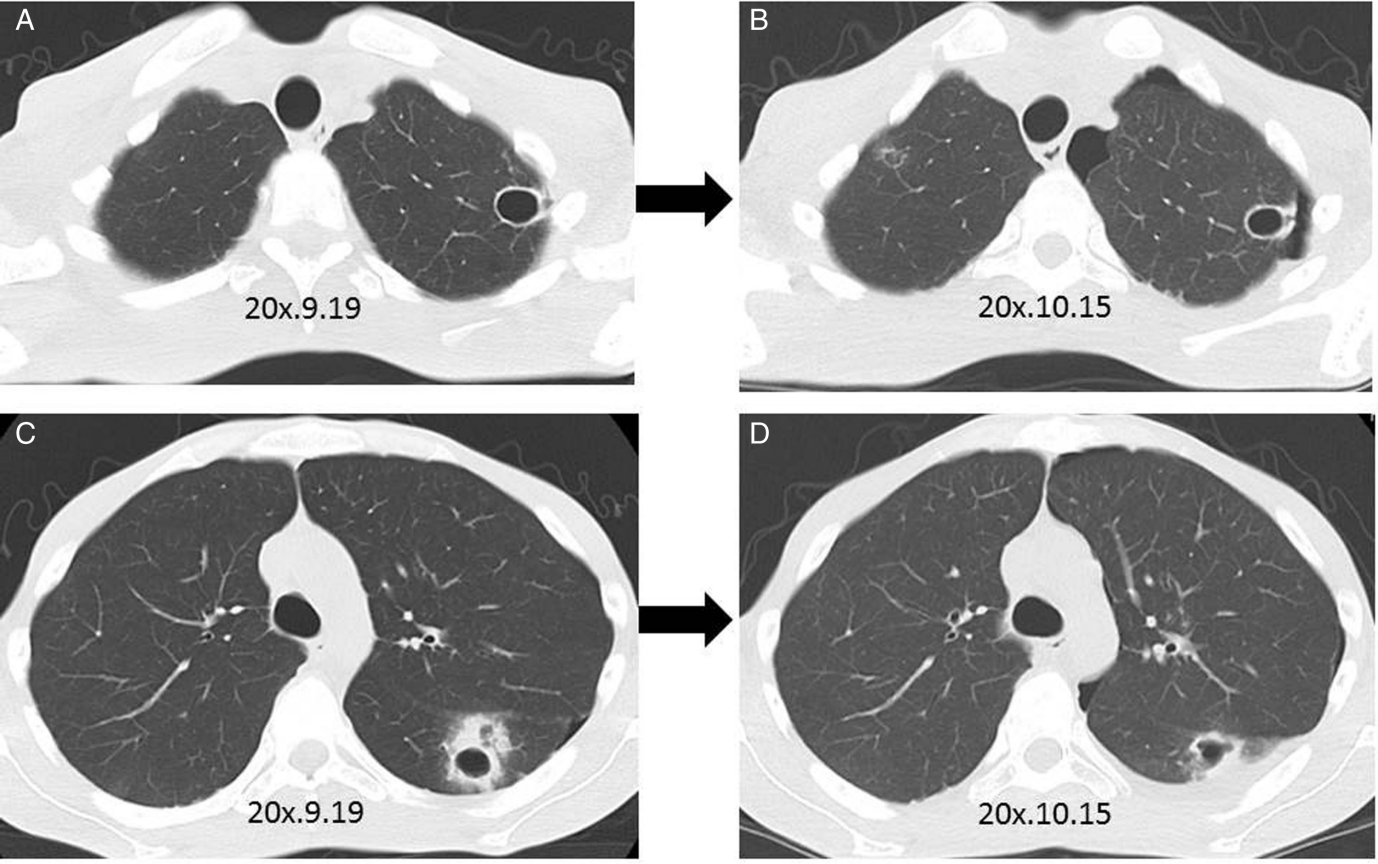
(A) Chest computed tomography shows a cavity in left upper field. (B) The cavity in left upper field is growing, adheres to the visceral pleura, and ruptures. A cyst in right upper field appears. (C) A cavity in left lower field is seen. (D) The cavity changes shape and adheres to the visceral pleura.
